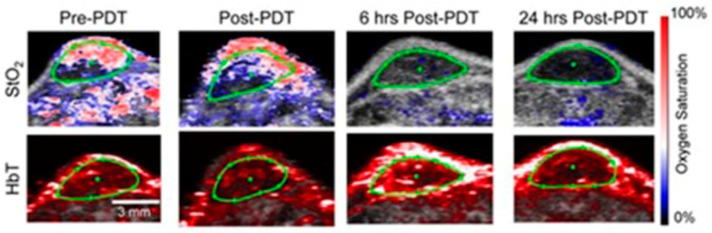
Combined ultrasound and photoacoustic images of responders in 1 h post-injection of photosensitizer before and after (immediate, 6 h, and 24 h) photodynamic therapy (PDT). (Top row) Ultrasound image in grayscale overlaid with oxygen saturation (StO_2_) map where blue and red represent hypoxic and oxygenated regions, respectively. (Bottom row) Ultrasound image in grayscale overlaid with total hemoglobin image (HbT). The green circle indicates the tumor region identified using ultrasound image. Adapted with permission from Mallidi, S.; Watanabe, K.; Timerman, D.; Schoenfeld, D.; Hasan, T. Prediction of tumor recurrence and therapy monitoring using ultrasound-guided photoacoustic imaging. *Theranostics*, 2015, *5*, 289 [250].
